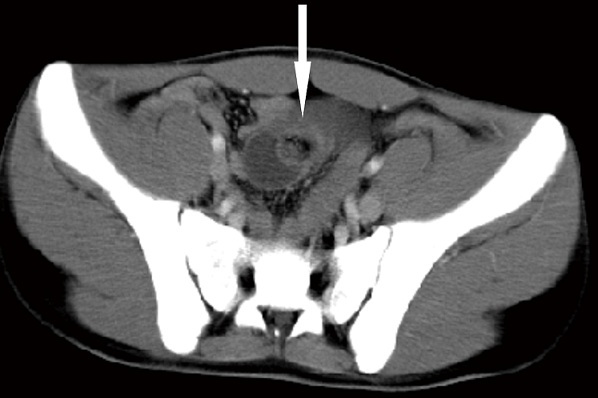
Typical sign of intestinal intussusception in abdominal CT scan: concentric circle (white arrow)
